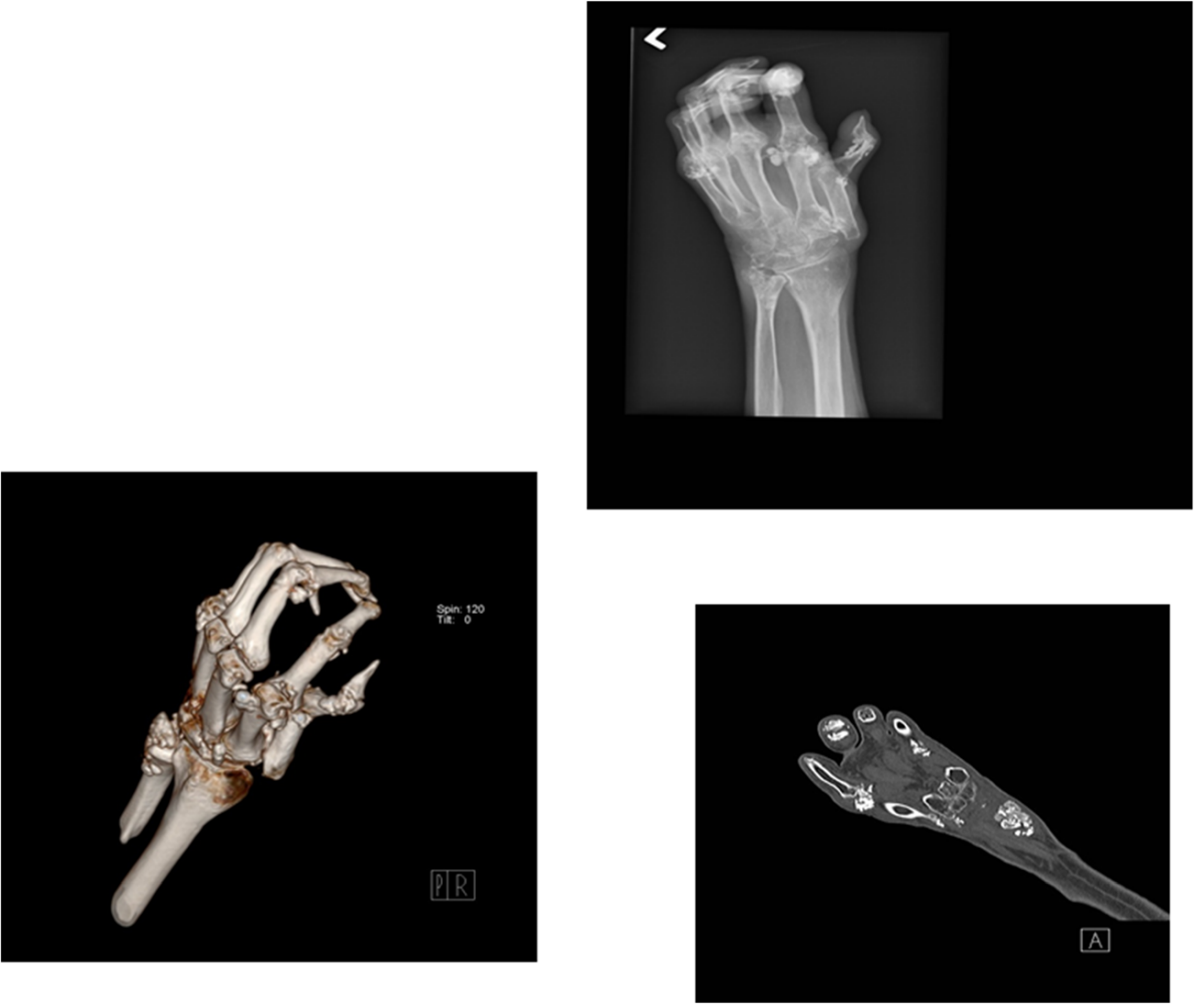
Oblique volume rendered 3-D CT, a single image from the coronal CT with bone windows, and an oblique radiograph of the left hand are shown: CT imaging shows better views of the extensive calcinosis surrounding the carpal bones, distal ulna, metacarpophalangeal (MCP) joints, and interphalangeal joints and extensive periarticular erosions with destructive changes of the articular cortical surfaces. CT sections show some calcinosis deposits deep to the tendons, others are attached to tendons. There are flexion deformities of all fingers and diffuse subluxations involving the MCP joints and first carpometacarpal joint
